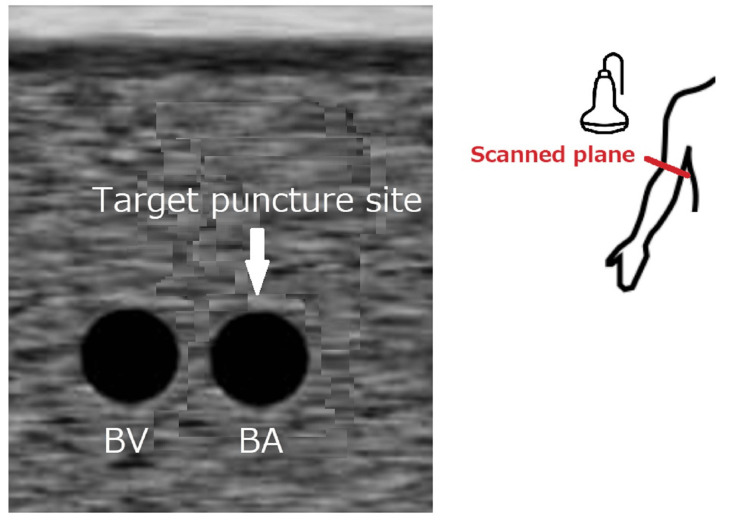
Scheme of the ultrasound image The diameters of the two vessels were nearly identical, and vascular compressibility on manual pressure application was confirmed on both vessels. BA: brachial artery; BV: brachial vein
